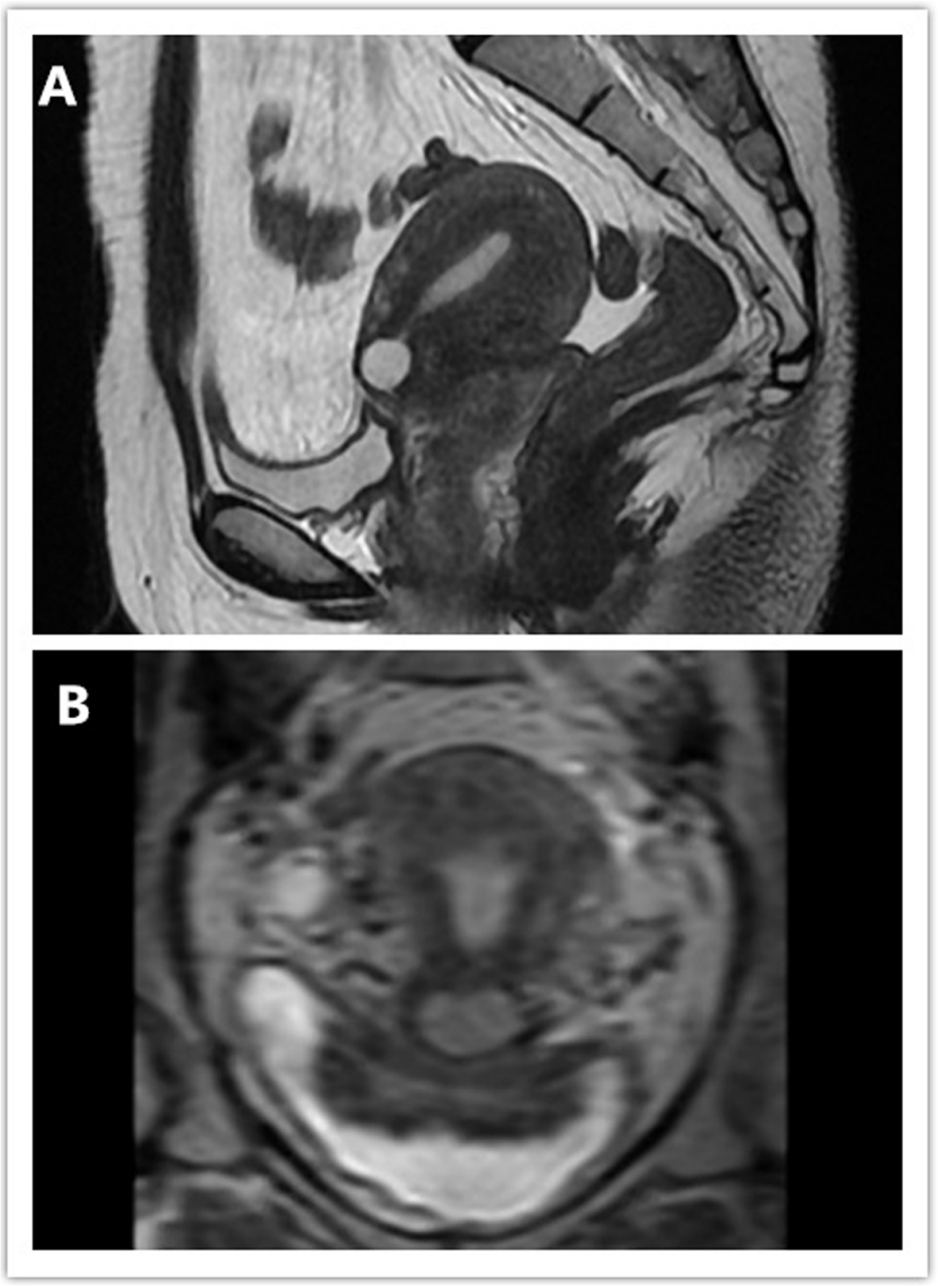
MRI images of cesarean scar defects. A. Sagittal view on T2 images. B. Coronal view on T2 images
